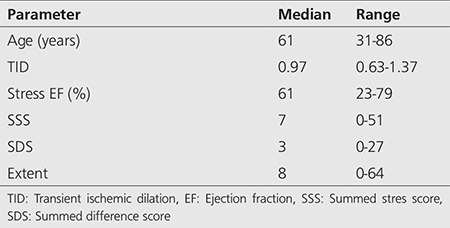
Demographic characteristics and myocardial perfusion scintigraphy gated single-photon emission computed tomography parameters
